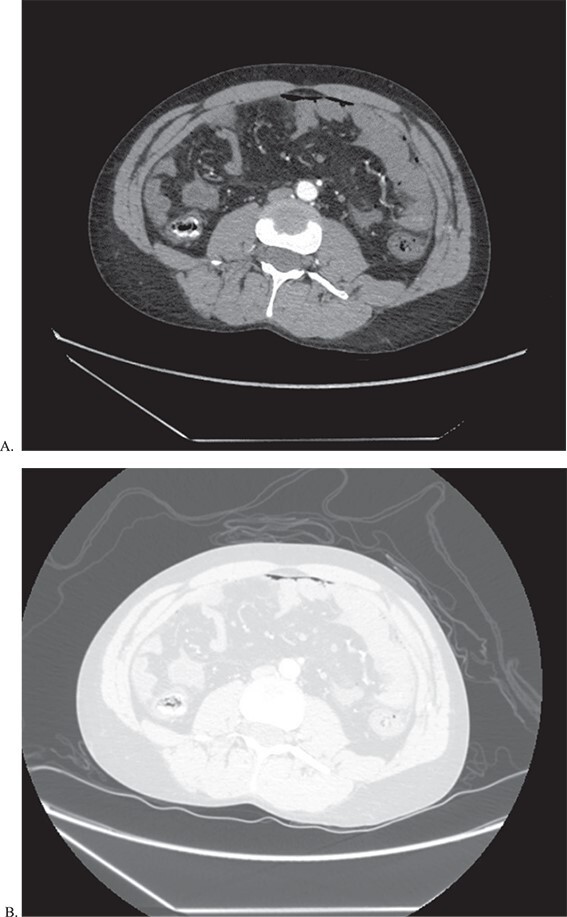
CT of abdomen and pelvis in both abdominal (A) and lung (B) window to show pneumoperitoenum as well a thickened loops of bowel.
